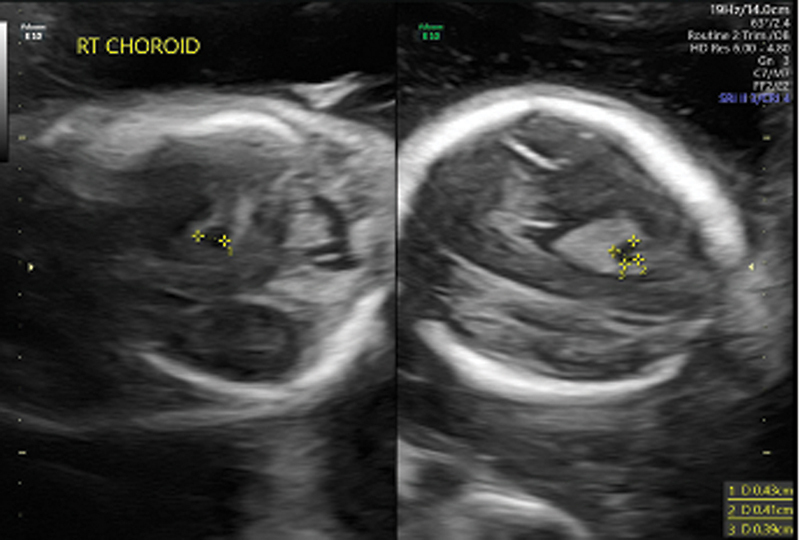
Ultrasound images at gestational week 20 showing right choroid plexus cyst measuring 0.43 × 0.41 × 0.39 cm.
